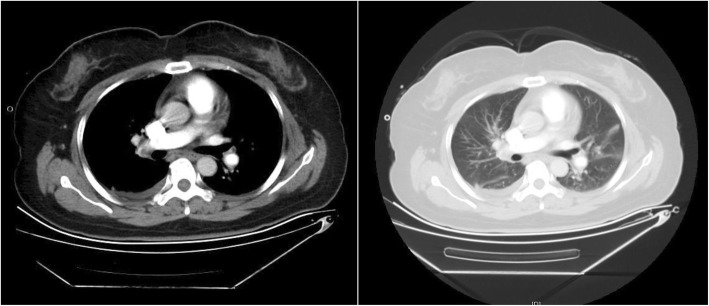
Computed tomography pulmonary angiography (CTPA) revealing no evident improvement of the thrombosis in the right and left main pulmonary artery, but a disappearance of the right pleural effusion
